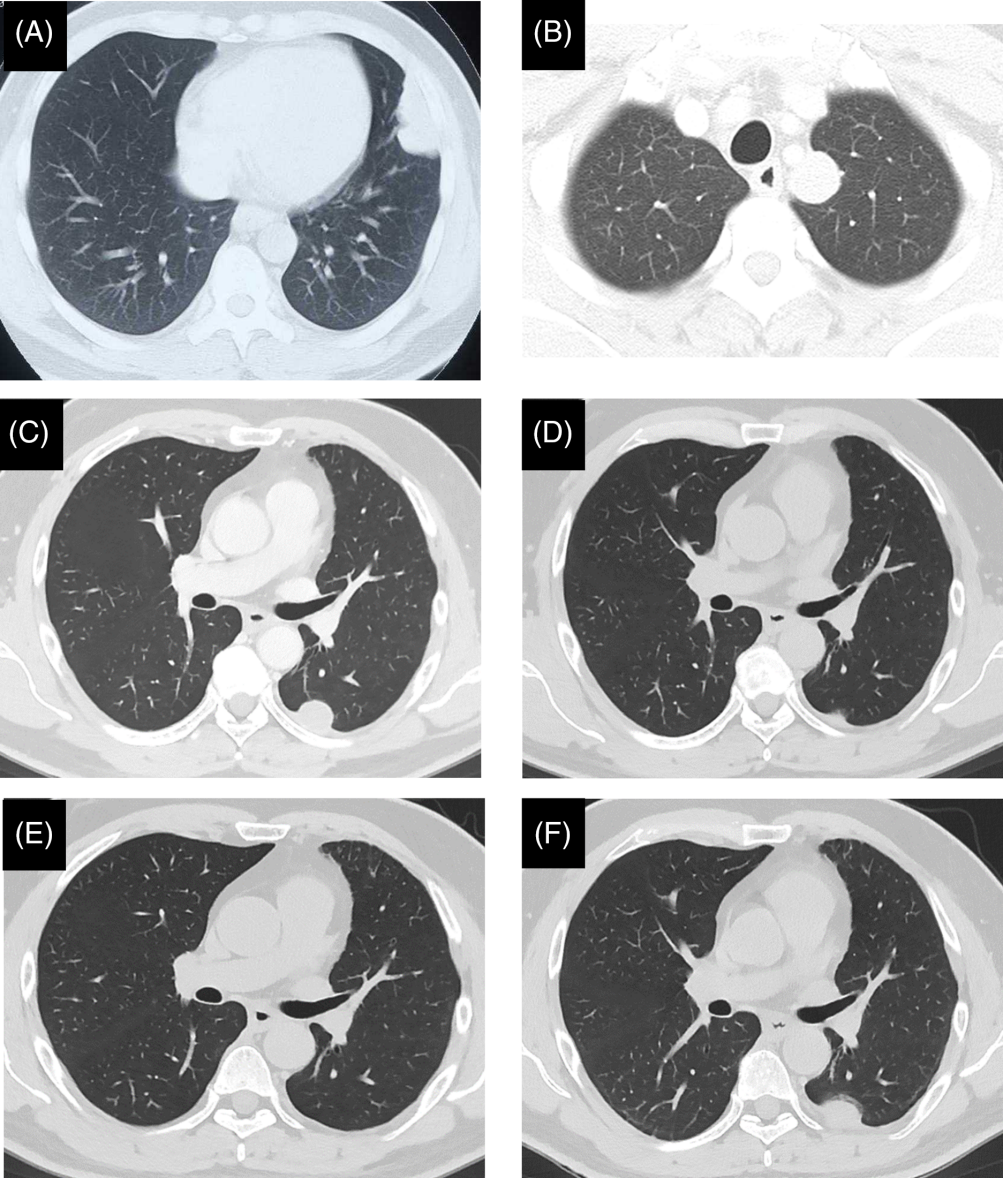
Chest computed tomography at the time of the first surgery revealed a nodule arising in the left pleura (A). Chest computed tomography at the time of the second surgery revealed a nodule adjacent to the mediastinum in the left upper hemithorax (B). Chest computed tomography revealed a nodule arising in the pleura adjacent to the left lower lobe three years after the second surgery (C). The tumour spontaneously regressed one month after C (D) and almost disappeared three months after D (E). However, the tumour increases in size again and gradually enlarged 18 months after E (F).
